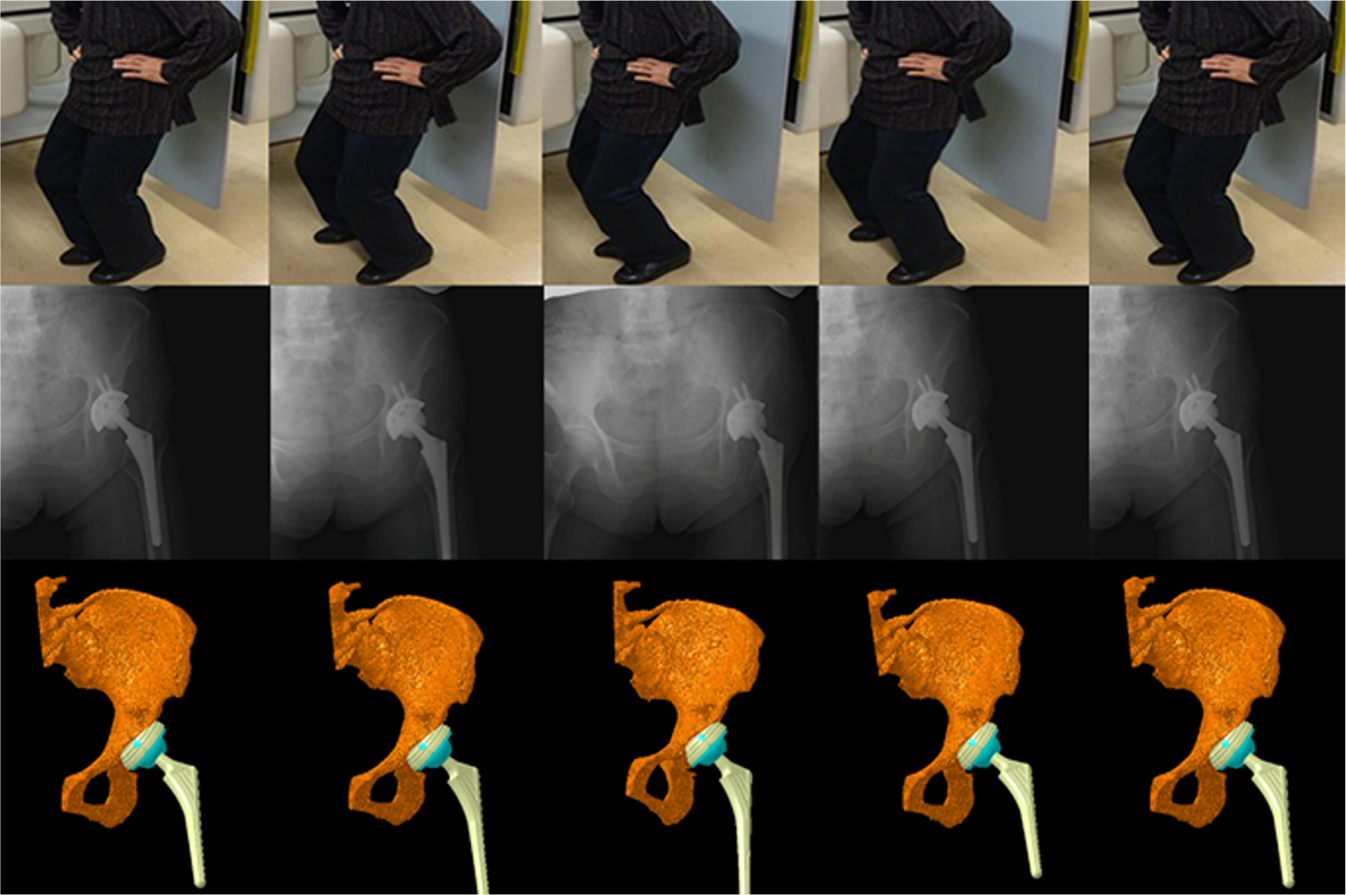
The hip motions during the hula dance movement kaholo were captured as continuous X-ray images using a flat panel X-ray detector (*middle stand*) to reconstruct three-dimensional images of the replaced hip joint (*lower stand*) using image-matching techniques
